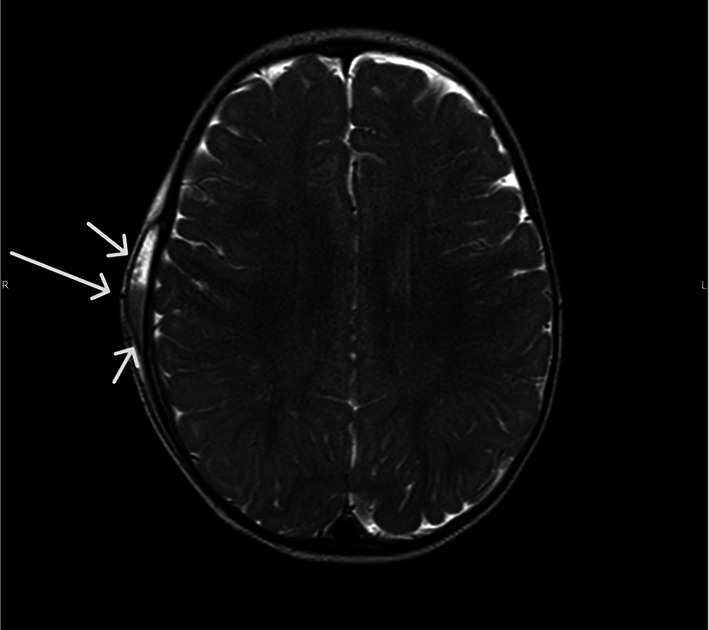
T2-weighted TSE Brain MRI in the transverse plane reveals subdural blood in the right parietal area (short arrows), with minimal imprint on the adjacent parenchyma. A compound fracture of the right parietal cranial theca is also observed (long arrow)
